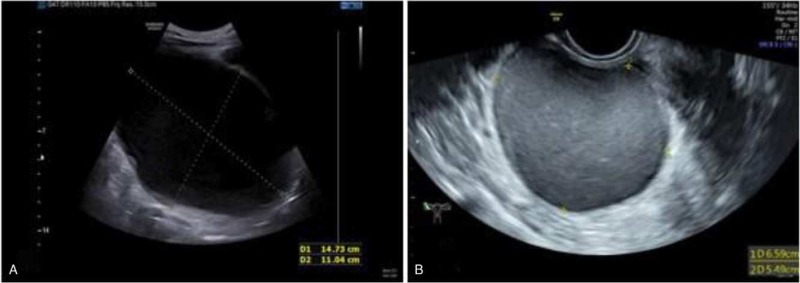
Abdominal ultrasound notes an anechoic black area measuring 6 cm, considering an endometriosis cyst a month ago (A), a huge pelvic mass measuring 15 cm at the patient's second visit (B).
